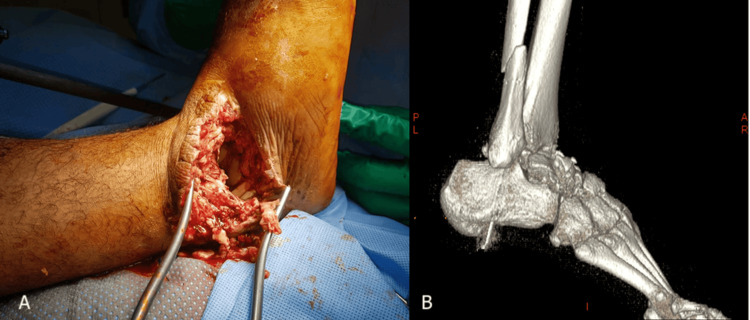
(A) Intraoperative and (B) 3D CT image of the lateral aspect of right foot with intraoperative image of fracture dislocated right talus.
